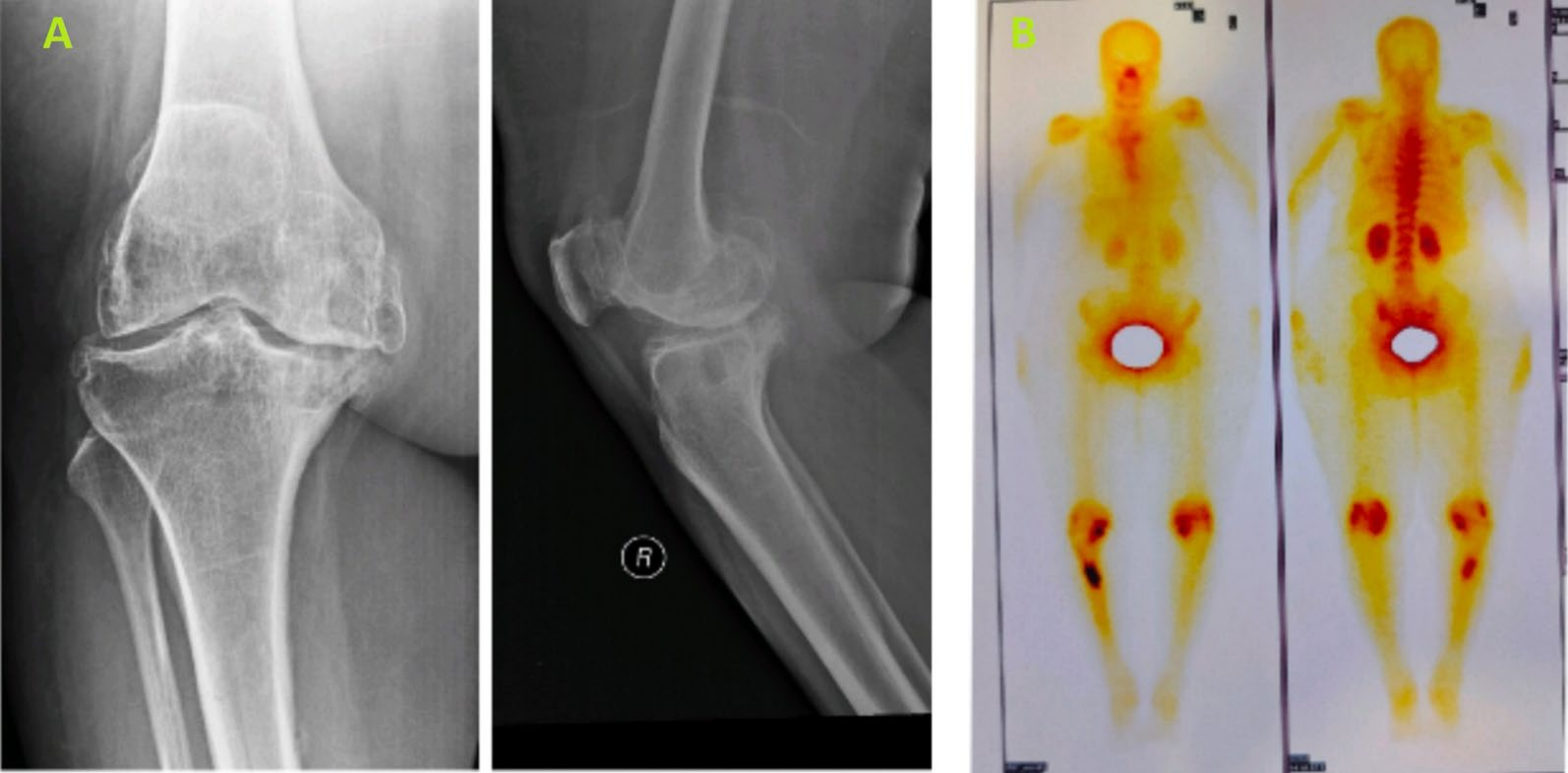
A On the left, preoperative radiograph of case 2 showing grade 4 osteoarthritis of the knee. The patient also had shin tenderness but no clear fracture line on the radiographs. B On the right, nuclear bone scan of the case 2 patient showing diffuse extensive lesion in right tibia with highly increased activity in the corticomedullary region
